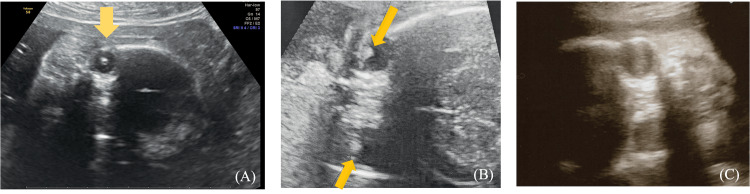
Fetal ultrasound image (25 weeks and three days in gestation). (A) Image from the frontal view of lens opacity highlighted with yellow arrows. (B) Image from the transverse view (scan) of lens opacity in both eyes highlighted with yellow arrows. (C) Fetal ultrasound image showing normal ultrasound findings at 26 weeks gestation as a reference image (25 weeks three days gestation). Normal lenses show white circles with a black center.
